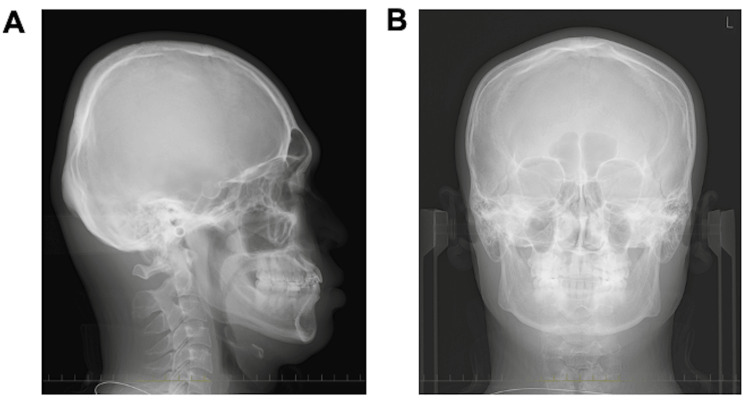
Cephalography performed six years after the retention treatment A: lateral cephalometric radiography obtained six years after the retention treatment, B: frontal cephalometric radiography acquired six years after retention treatment
